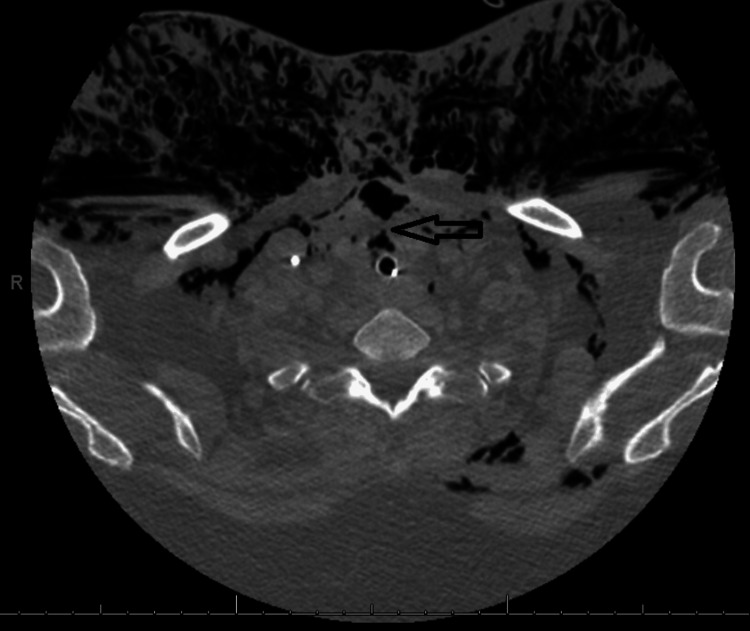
CT neck showing possible focal defect in the anterior tracheal wall (black arrow)
